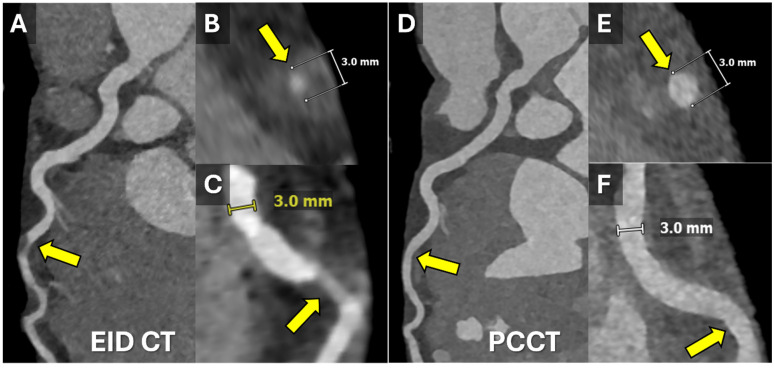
Use of Energy-Integrating and Photon-Counting Detectors. CCTA images from a 38-year-old woman with SCAD affecting the mid-LAD. The SCAD was first detected on a CCTA performed on a CT scanner with a standard energy-integrating detector (EID, (A–C)). The segment affected is indicated with arrows. A 1-month follow-up CT performed on a CT scanner with photon-counting detectors (PCCT, (D–F)) demonstrated spontaneous resolution of the SCAD (arrows). The minimum slice thickness on the EID CT is 0.6 mm compared to 0.2 mm on the PCCT. Note the improved spatial resolution (decreased blur) of PCCT, especially on the zoomed-in images ((B,C) vs. (E,F)). A 3 mm measurement on images is for purposes of scale. This improved spatial resolution makes it easier to detect subtle coronary lesions and significantly reduces blooming artifacts from coronary stents and heavily calcified atherosclerotic plaques. Abbreviations: CCTA = coronary computed tomography angiography, LAD = left anterior descending artery, SCAD = spontaneous coronary artery dissection.
